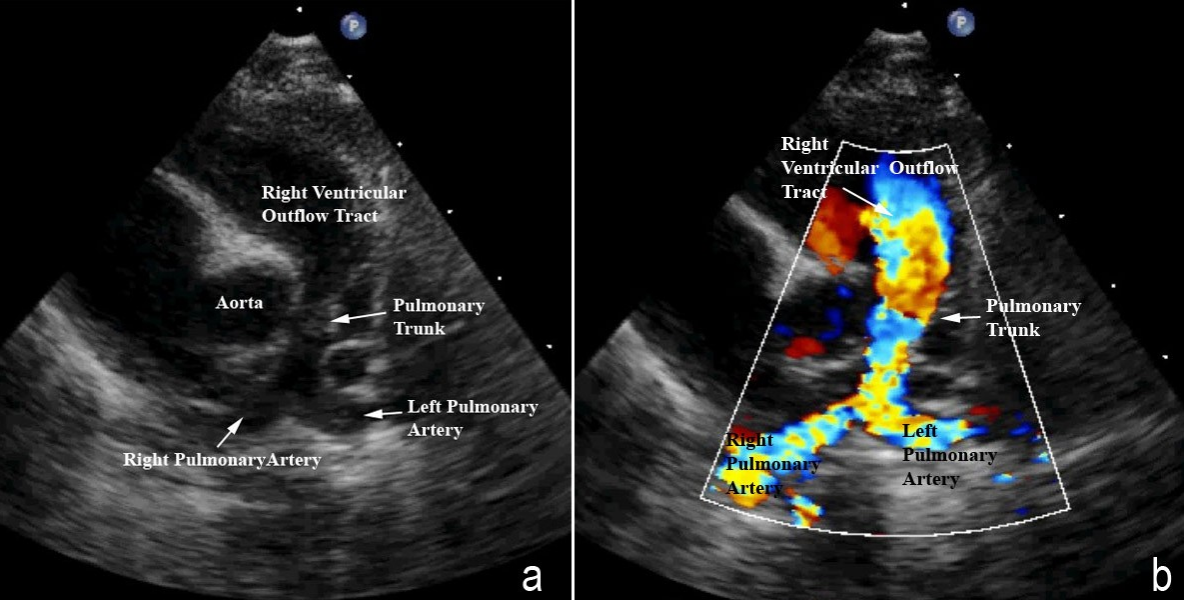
A slightly modified view (a), angled to optimize imaging of the pulmonary arteries in the patient imaged to produce Figure 6, reveals significant hypoplasia of the pulmonary trunk and the pulmonary arteries, which result from the antero-cephalad deviation of the outlet septum. The pulmonary valvar leaflets are not visualized. In panel b, colour Doppler has been used, and demonstrates turbulence and acceleration of the flow of blood in the right ventricular outflow tract, originating at the level of the deviated outlet septum. The turbulence continues into the hypoplastic pulmonary trunk and pulmonary arteries.
